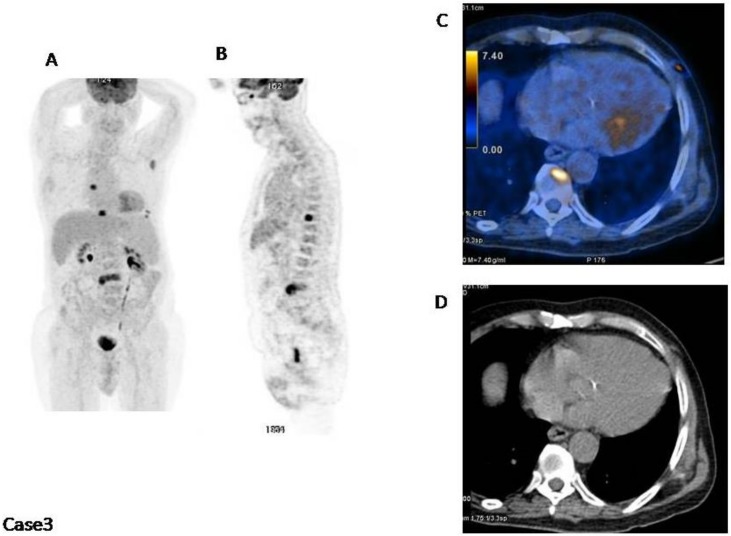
(A) MIP; (B) PET sagittal cut; (C) fused images axial cut; (D) CT axial cut. There are at least four focal hot spots with lytic lesions underlying and a subcutaneous lesion in the left thorax (C).
